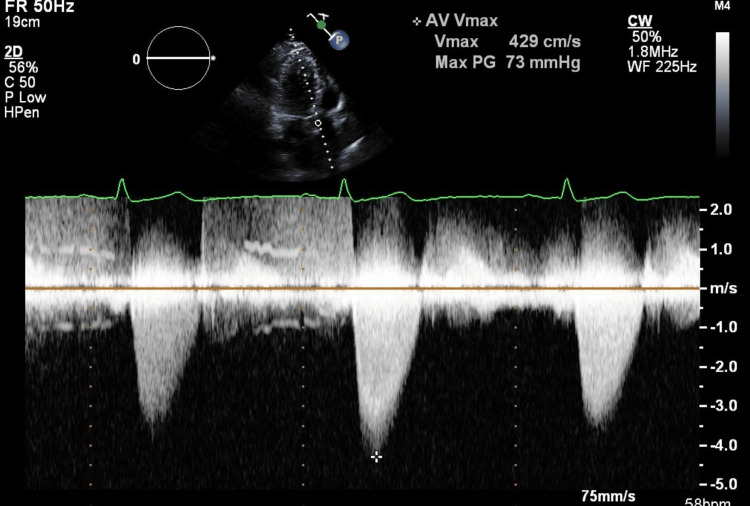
Transthoracic echocardiogram (apical 3 chamber view) with Doppler measurements across the aortic valve, demonstrating peak velocity of 4.29 m/s.
